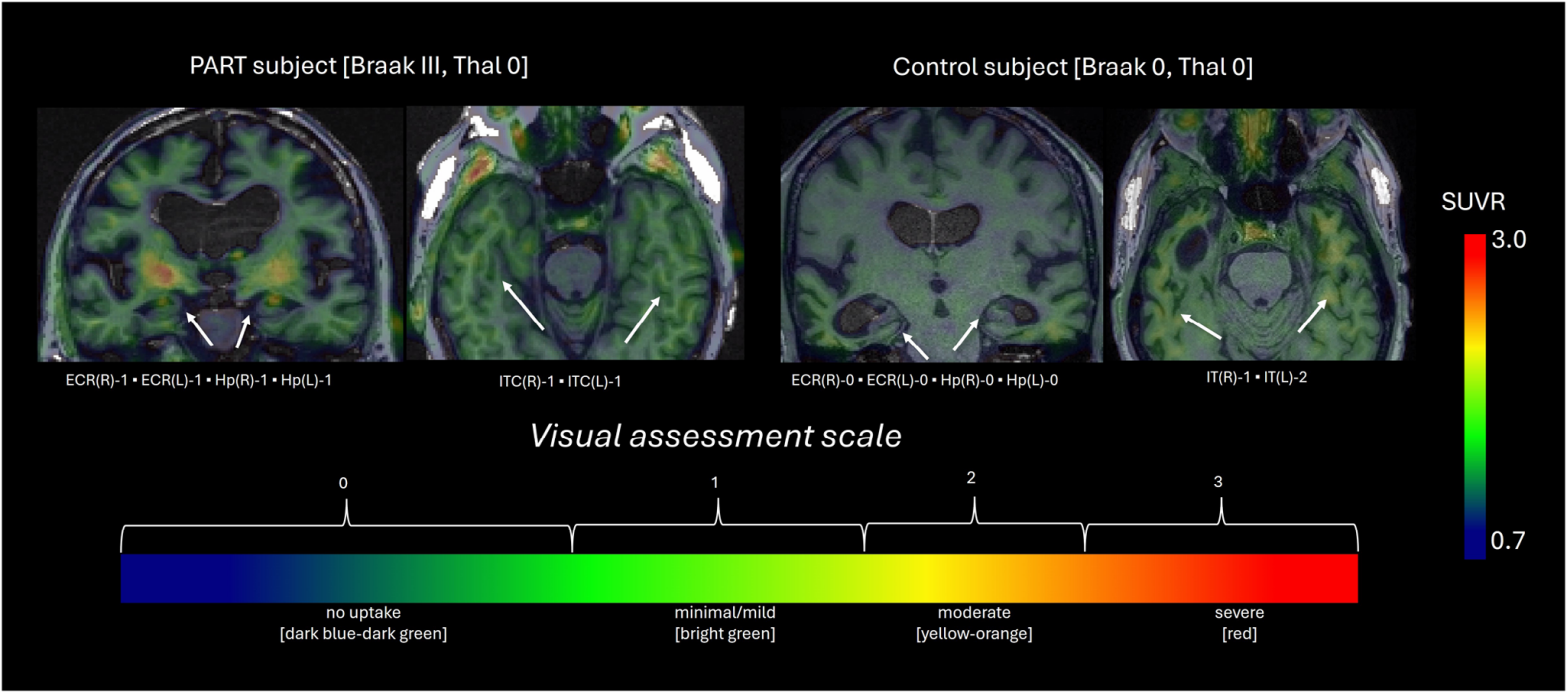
Visual grading scale used for ^18^F‐flortaucipir (FTP) PET images assessment. Upper row displays exemplary FTP‐PET scans in PART (Braak III/Thal 0) and control (Braak 0/Thal 0) subjects. Yellow arrows indicate regions assessed for FTP uptake. ECR, entorhinal cortex; Hp, hippocampus; ITC, inferior temporal cortex; L, left; PART, primary age‐related tauopathy; PET, positron emission tomography; R, right; SUVR, standardized uptake value ratio.
